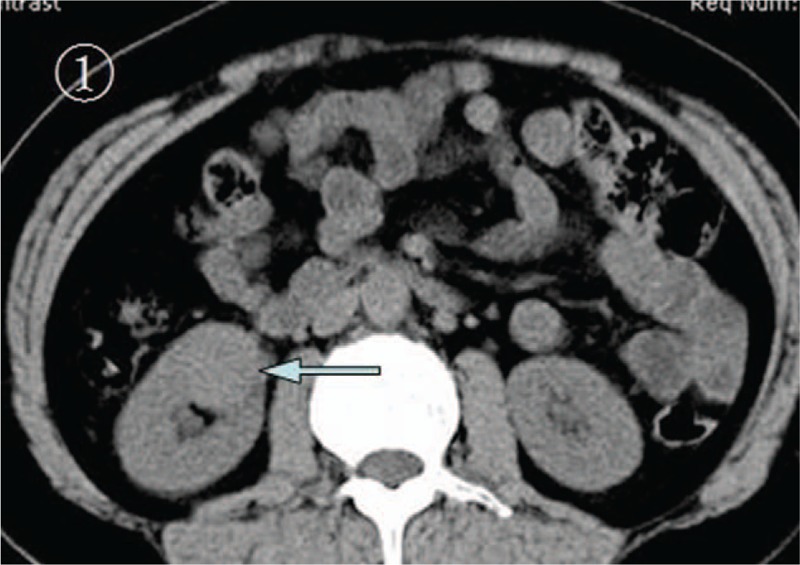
The abdominal precontrast CT scan. This scan revealed a mass that was measured about 3 cm at the hilum of the right kidney (arrow). CT = computed tomography.
